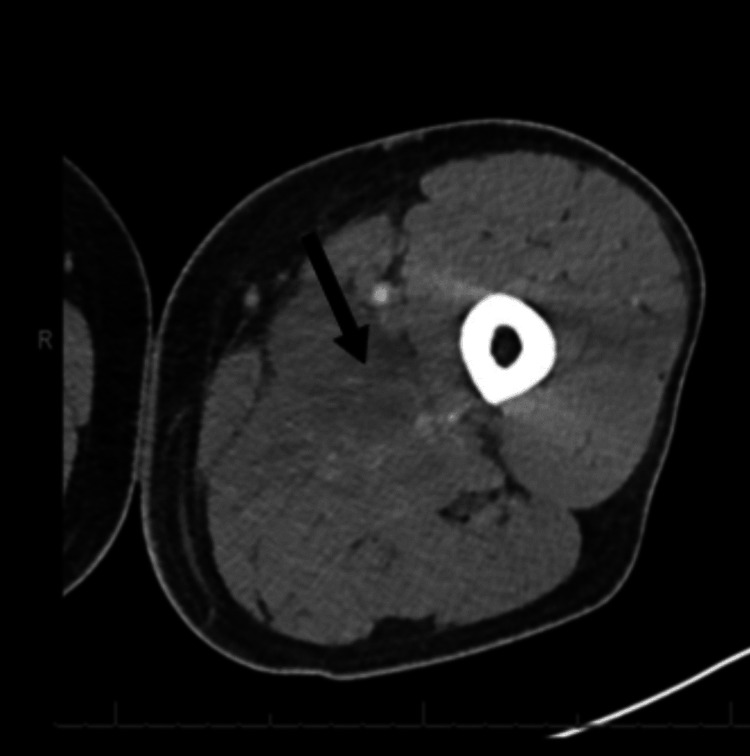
Transverse CT of the left lower extremity showing hypoattenuation of the adductor longus muscle within the medial compartment (arrow)
